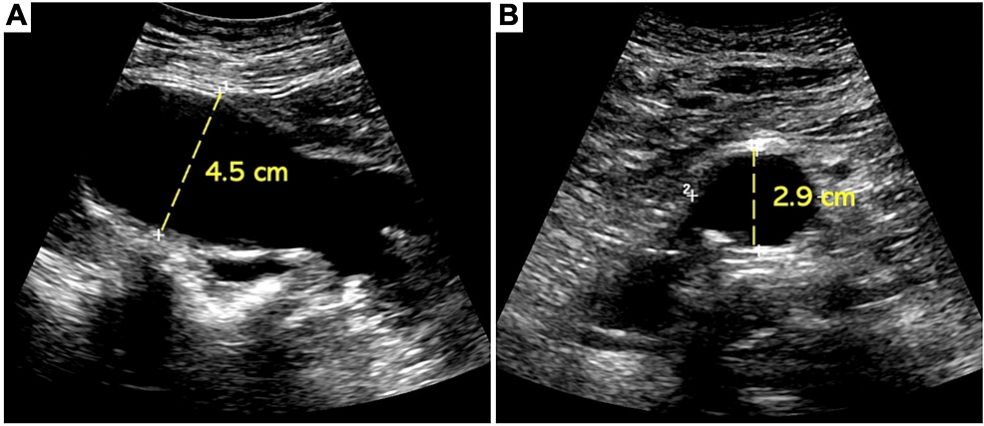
Arterial ultrasound of the abdominal aorta and left common iliac artery. (A) Aneurysm of the left common iliac artery approximately 10 years after its initial discovery, measuring 4.5 cm. (B) Infrarenal abdominal aorta with fusiform dilatation, measuring 2.9 cm.
